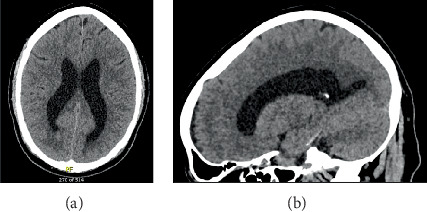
CT brain imaging demonstrating prominent ventricles. (a) Axial and (b) sagittal noncontrast CT images of the brain show prominent lateral and third ventricles without evidence of hydrocephalus, mass effect, or acute intracranial pathology. No signs of leptomeningeal disease were detected radiographically, despite the patient's neurologic symptoms (original image, Department of Medical Imaging, Western University).
